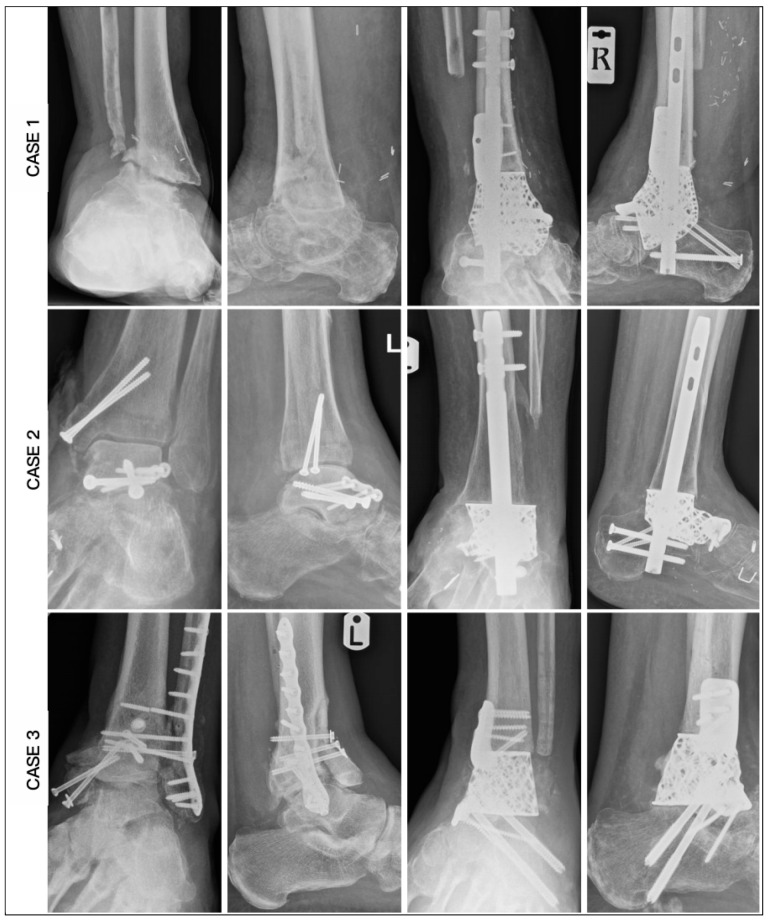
Plain radiographs of AP and lateral pre- and post-fixation for cases 1 to 3.
